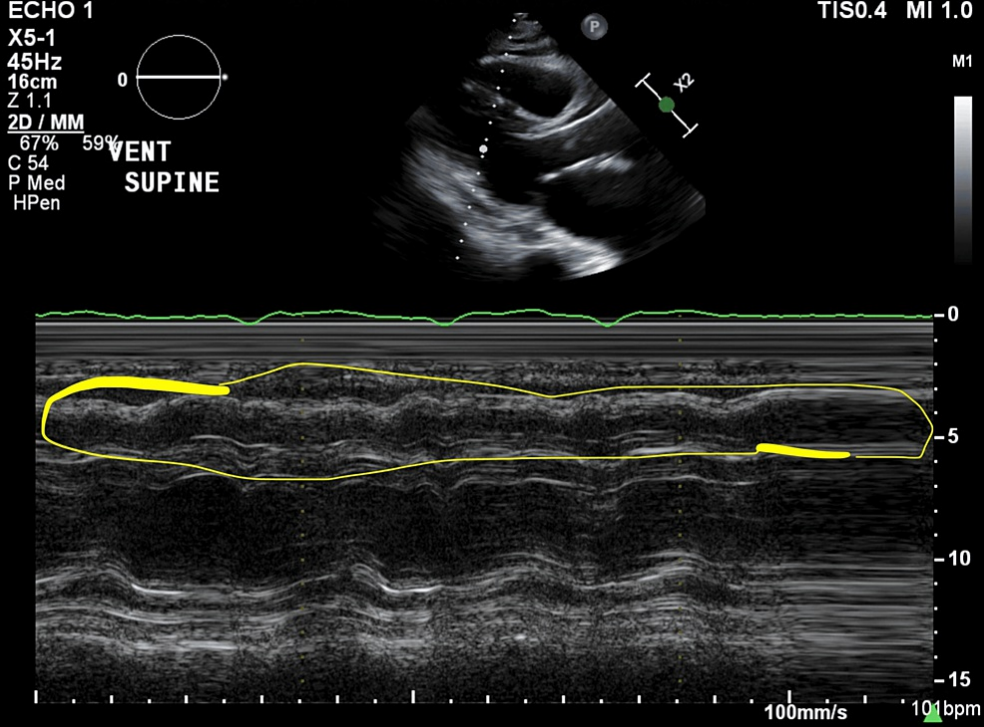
TTE image (parasternal long-axis view) The area circled shows a time-lapse image of the RV walls contracting and mild-moderate RV dysfunction. TTE: transthoracic echo, RV: right ventricular
